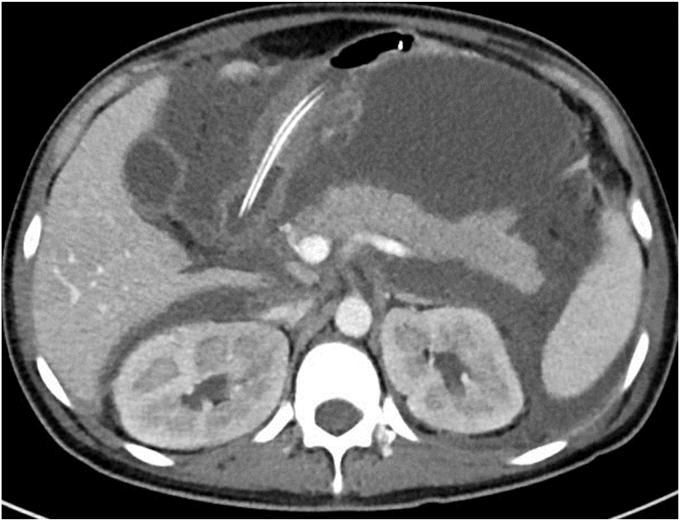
CT image at the time of admission. Inflammatory spillover throughout the abdominal cavity.
